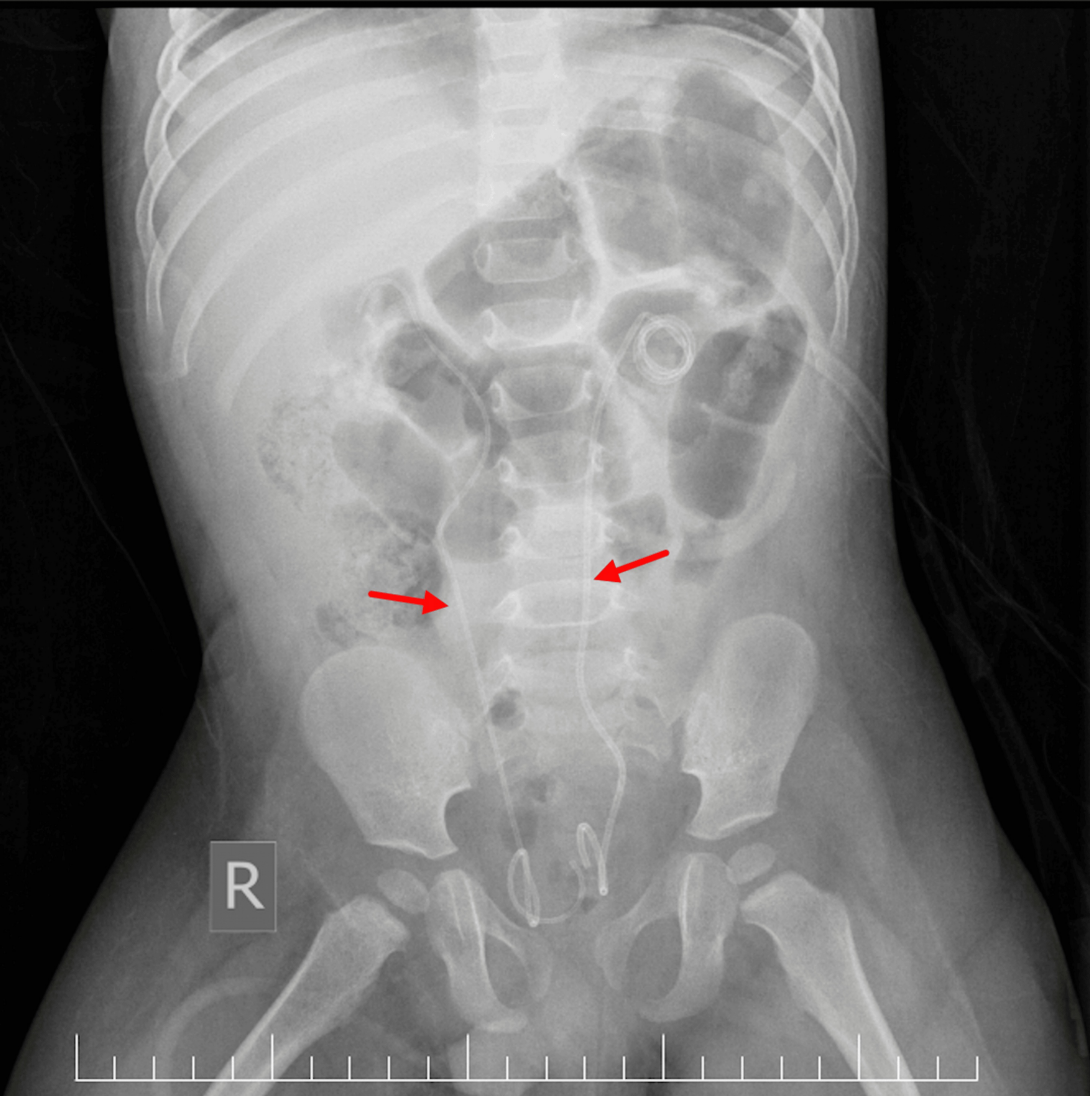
Post-cystoscopy abdominal X-ray Bilateral double-J (DJ) stents in place, demarcated by red arrows.
